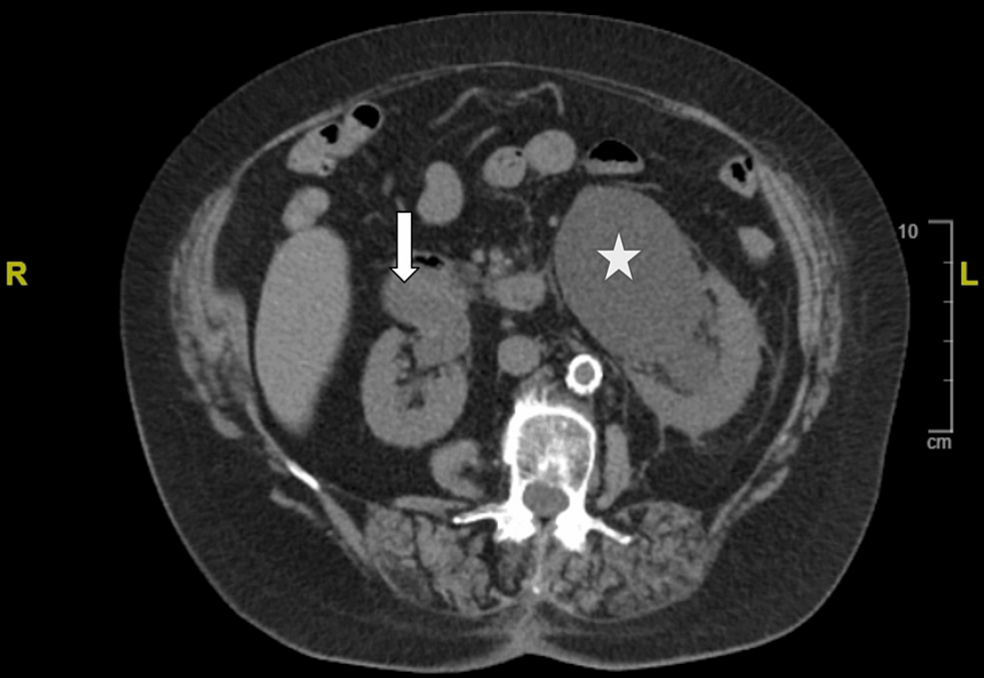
CT abdomen/pelvis without contrast in axial view shows bilateral hydronephrosis more prominent on the left (white star) compared to the right (white arrow).
